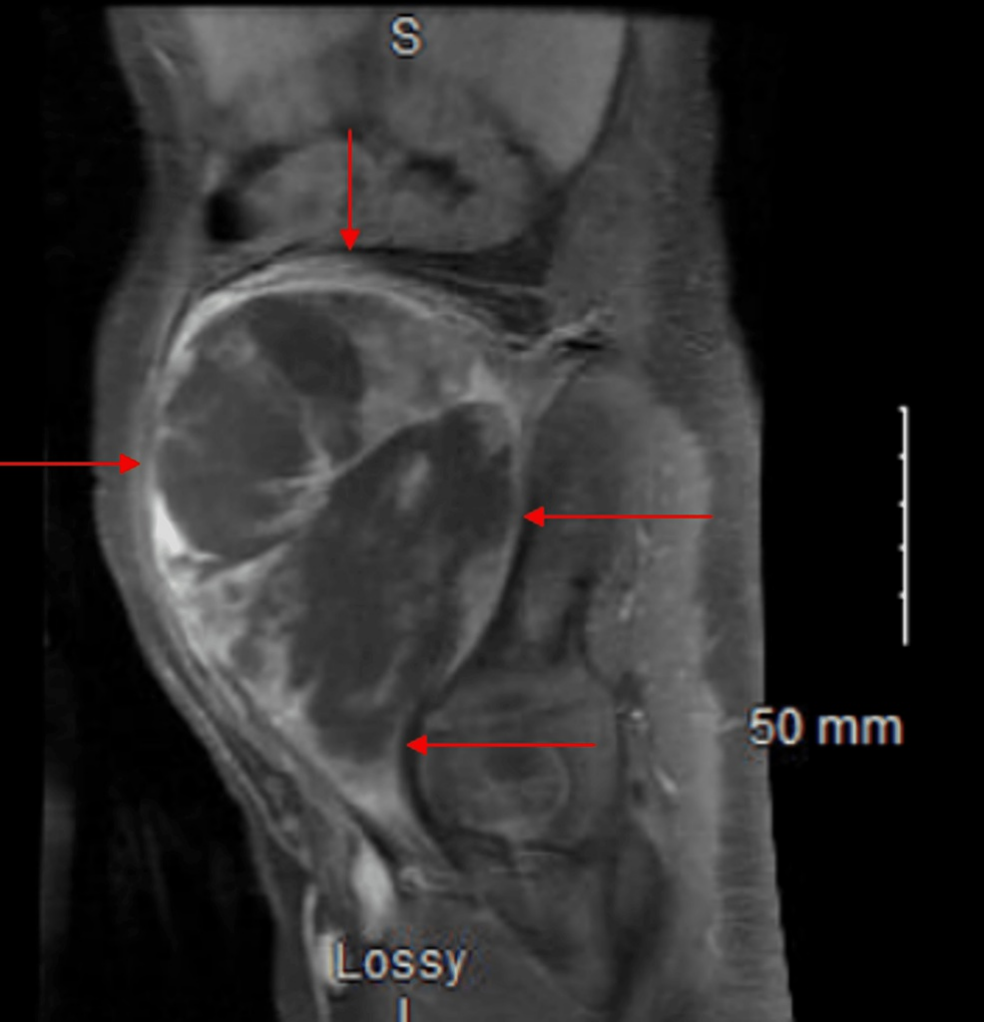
Sagittal T1-weighted MRI without contrast Rhabdomyosarcoma (RMS) is delineated by arrows in imaging.
